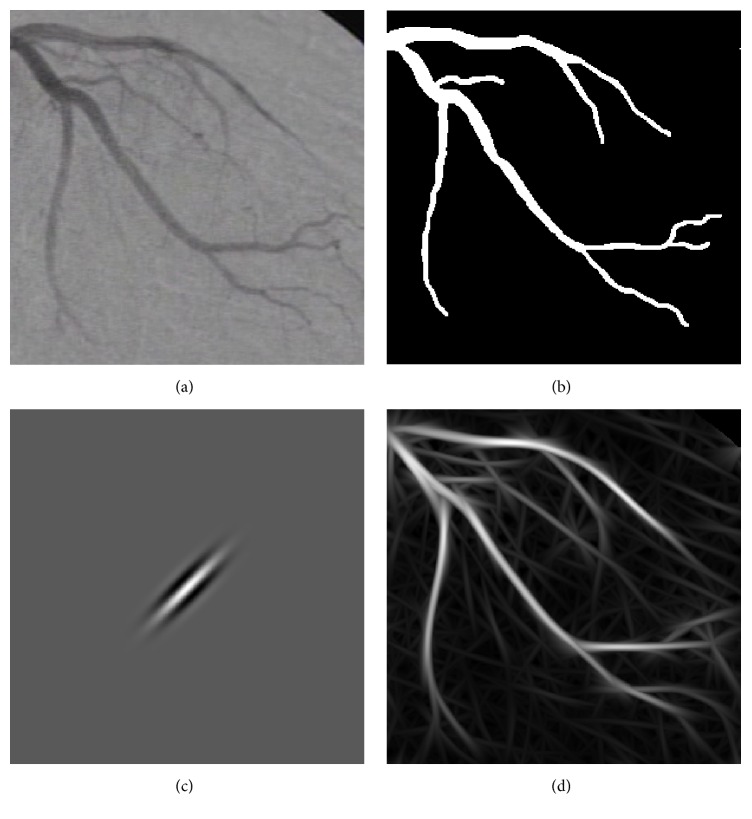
(a) Original X-ray coronary angiogram. (b) Ground-truth of angiogram in (a). (c) Gabor filter kernel with *l* = 2.9, *τ* = 15, and *θ* = 45°. (d) Resulting enhanced image using the angiogram in (a) and the filter bank of Gabor kernels with the parameters {*l*, *τ*} of (c), with *κ* = 180.
